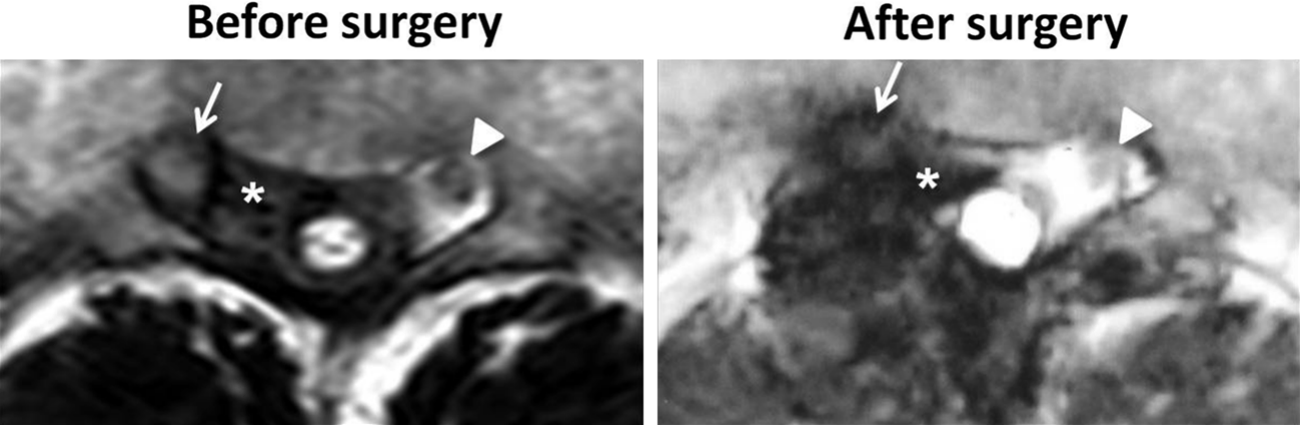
T2-weighted MRI of the sacral spine before and after surgery. The SOL (*asterisks*) seen before surgery is decreased and recognized only around the right S2 nerve root after surgery. *Arrows* and *arrowheads*, the *right* and *left* S2 nerve roots, respectively
